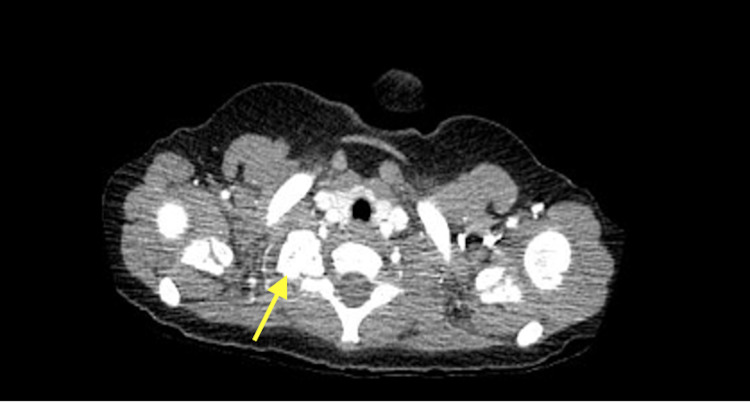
CT thorax with contrast showing osteochondroma (at yellow arrowhead) arising from the right first rib and possibly the transverse process of T1 1.8 x 1.8 x 1.6 cm calcified/ossified mass, appearing to arise from the right first rib, suggestive of osteochondroma. The almost complete calcification of this lesion makes the diagnosis of neuroblastoma less likely. CT - Computerized Tomography
